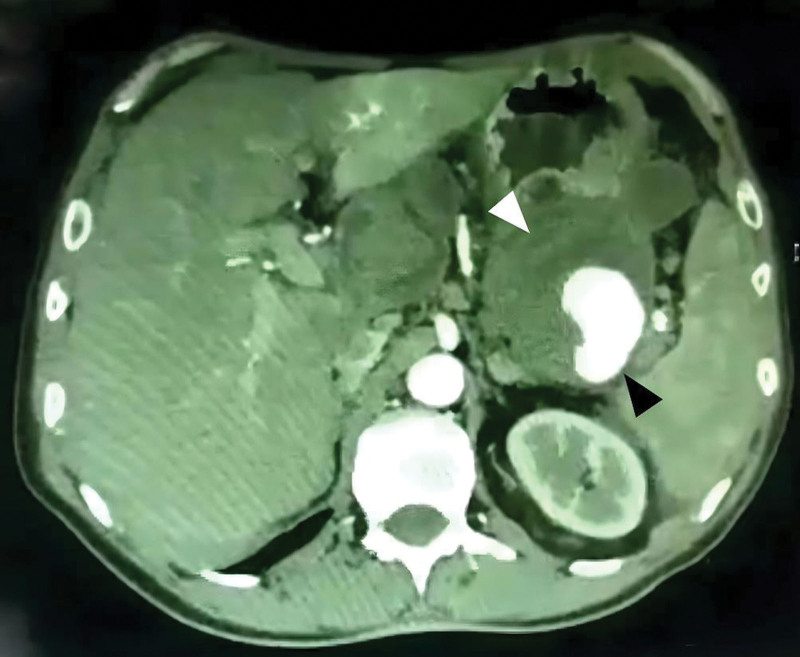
MSCT (cross-sectional image) shows a giant SAA with eccentric thrombosis (white arrow) and a true lumen (black arrow). MSCT = multi-slice computed tomography, SAA = splenic artery aneurysm.
